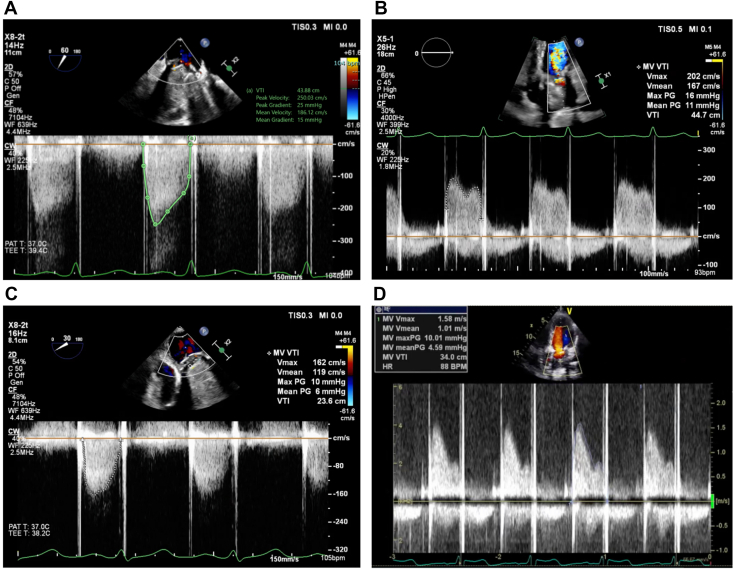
Serial 2D TEE- (A, C) and 2D TTE- (B, D) guided continuous-wave spectral Doppler profile displays of mean MV gradient over the course of fibrinolytic therapy representing the baseline findings (A, 15 mm Hg, 104 bpm), day 4 of treatment (B, 11 mm Hg, 93 bpm), day 10 of treatment (C, 6 mm Hg, 105 bpm), and day 14, end of fibrinolytic treatment (D, 5 mm Hg, 88 bpm).
